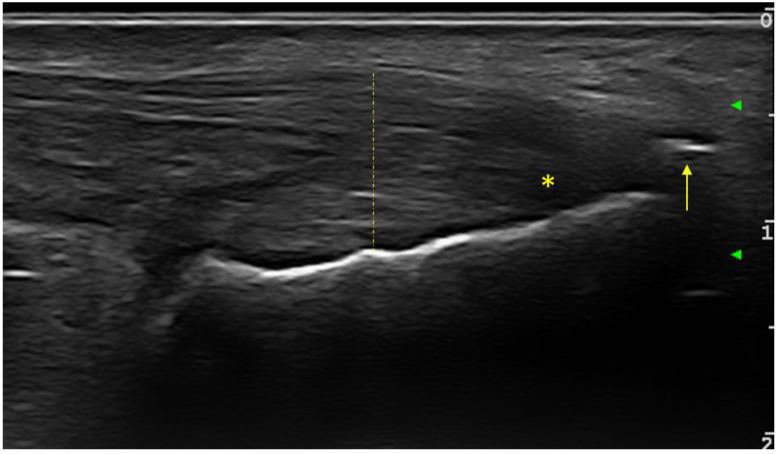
Longitudinal ultrasound scan of Achilles tendon OMERACT-defined enthesitis at calcaneus insertion in a PsA patient (grayscale examination): hypoechoic aspect (star), thickness increase (dashed line) and the presence of enthesophytes (arrow).
